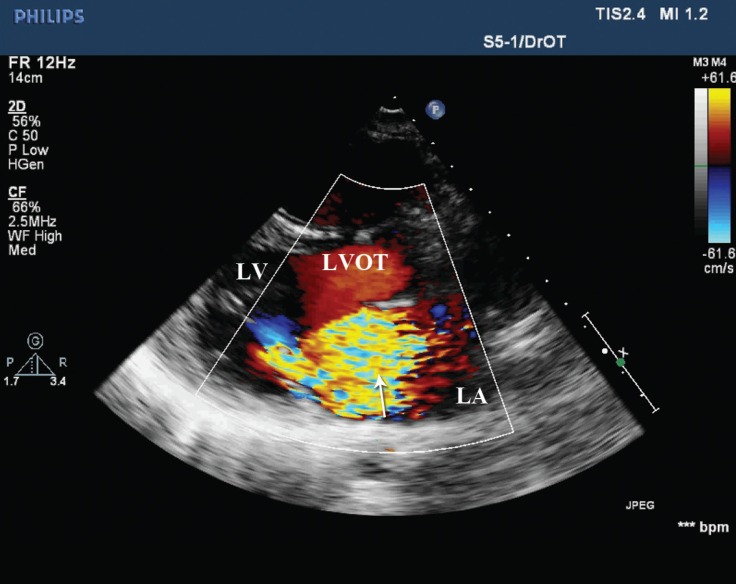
Parasternal long-axis echocardiographic view, showing severe mitral regurgitation (arrow)
